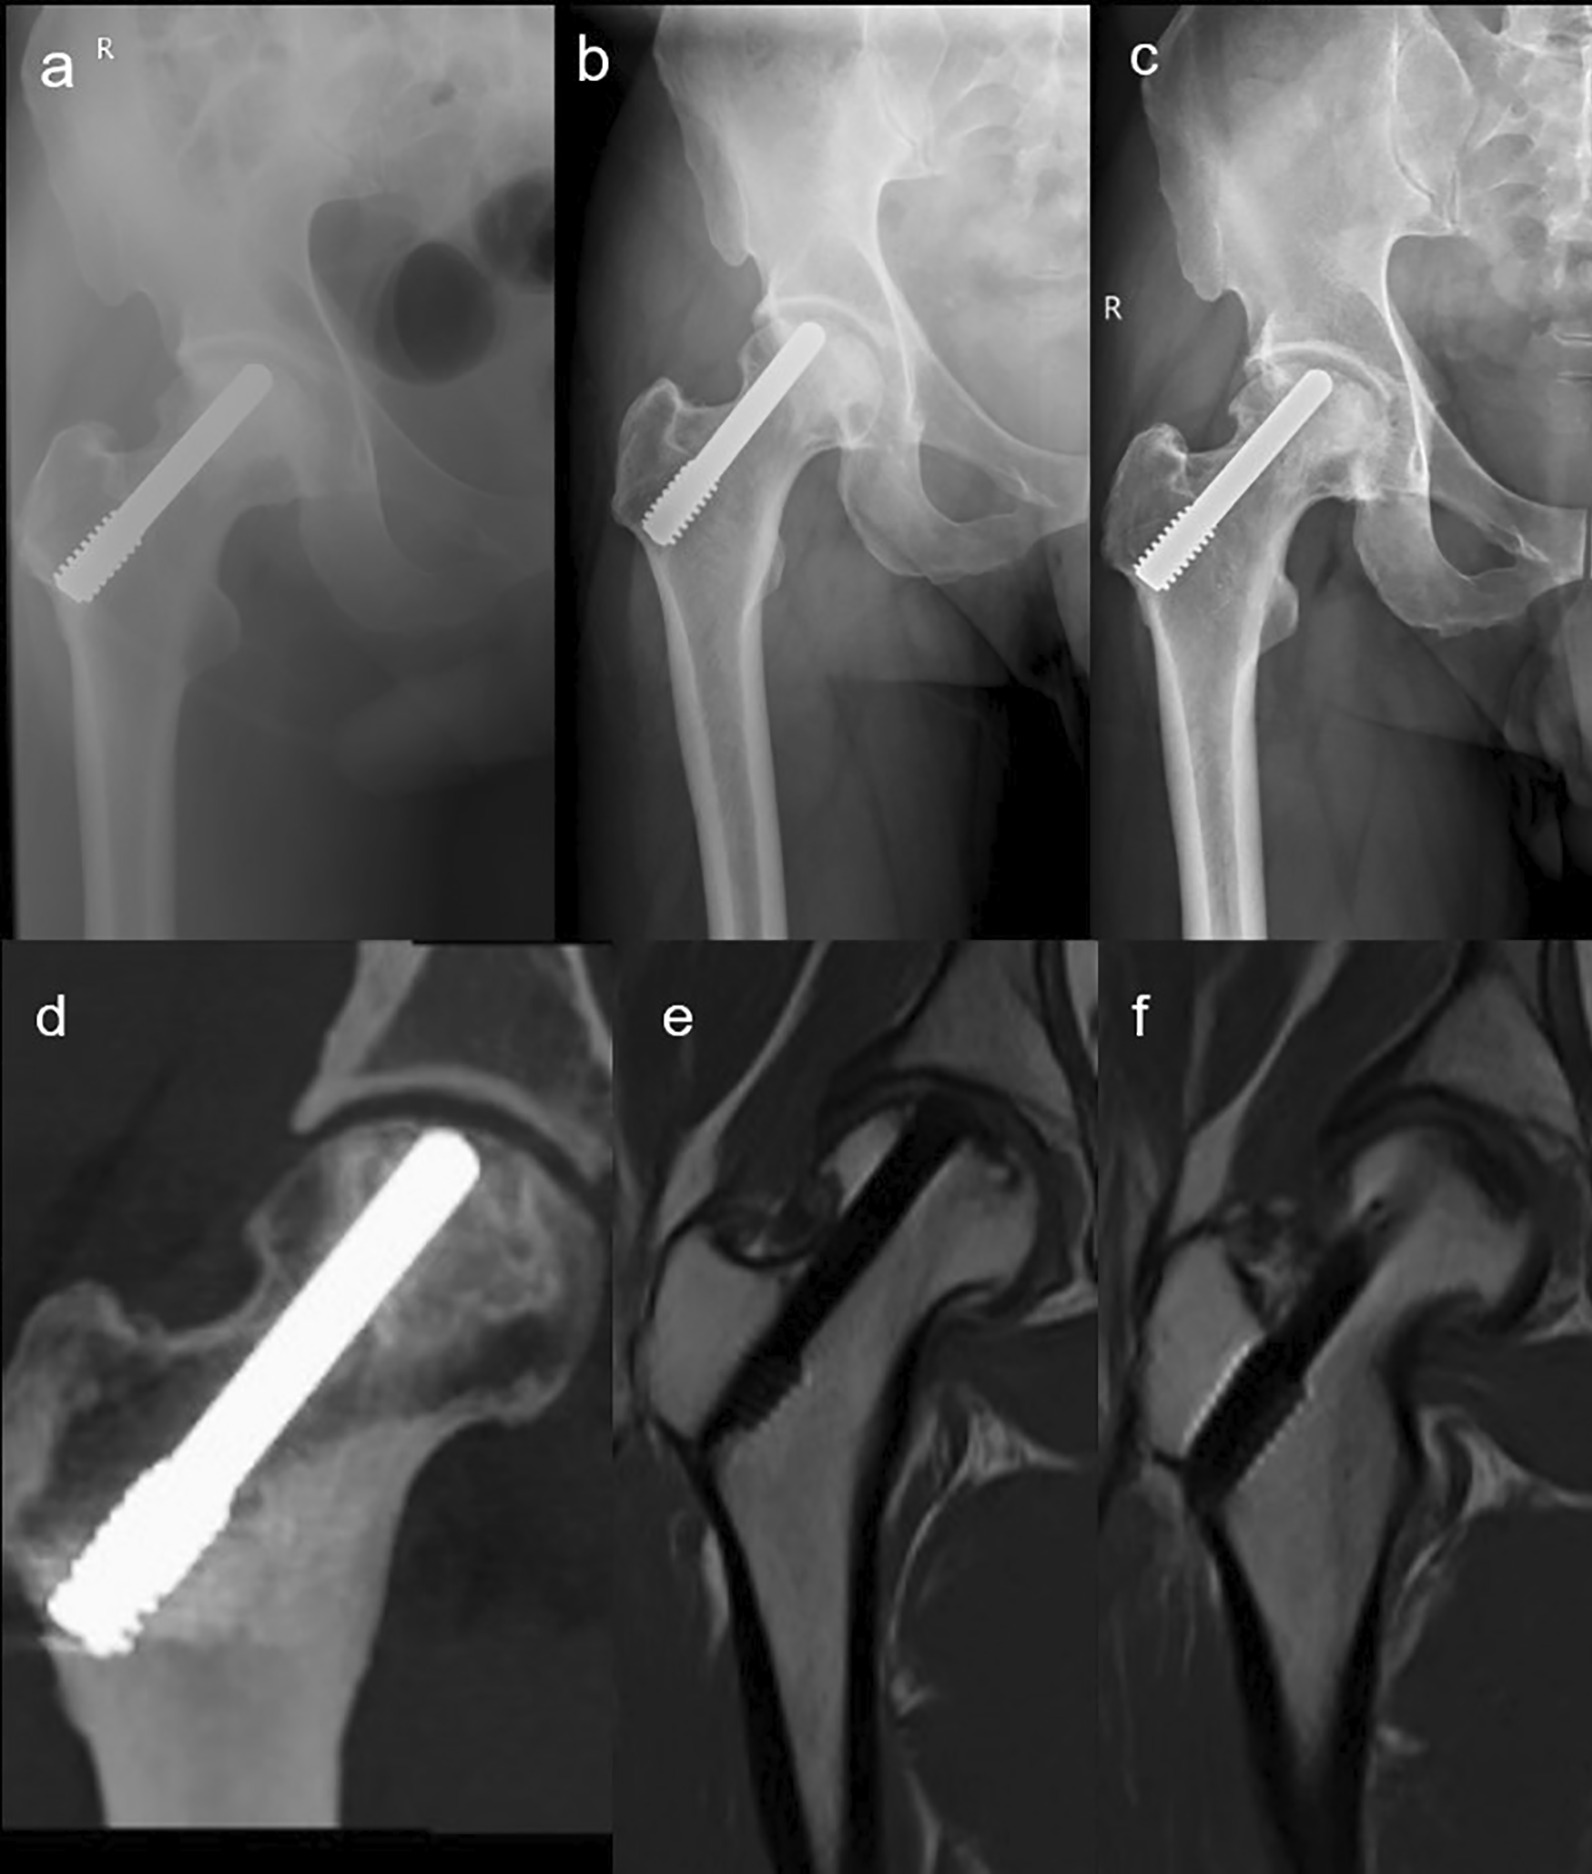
132 months follow-up of a patient with osteonecrosis of the femoral head (ONFH) a Postoperative X-ray; b 6 years postoperative X-ray; c 11 years postoperative X-ray; d 6 years postoperative CT; e, f 11 years postoperative MR
